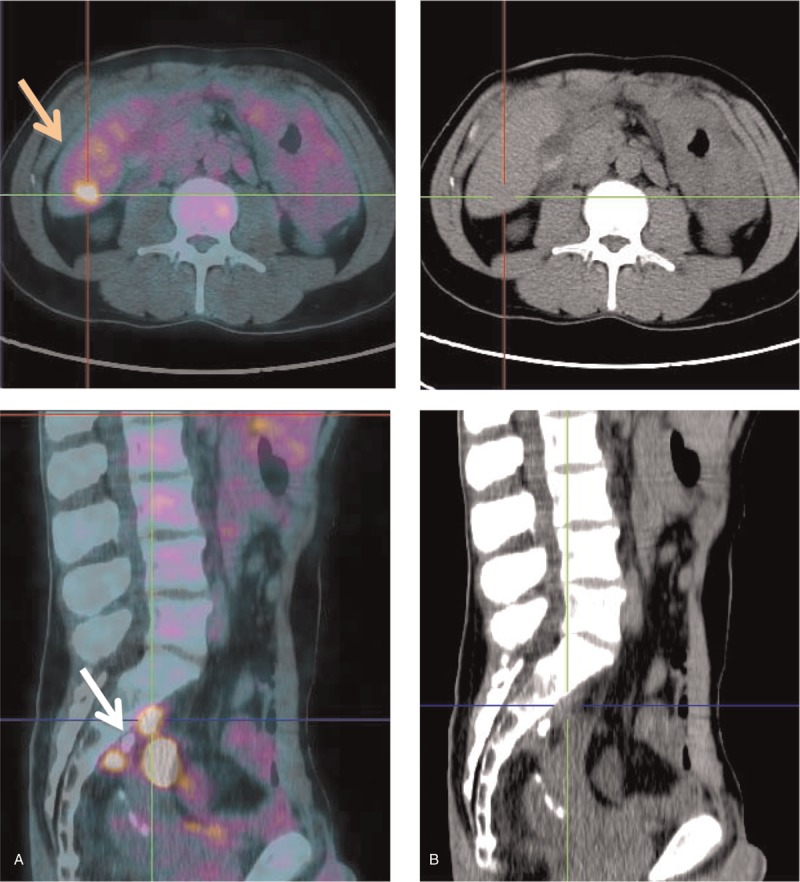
Axial (A) and sagittal (B) images: fused FDG-positron emission tomography/CT (left), CT alone (right). (A) New hepatic metastatic lesions (orange arrow). (B) Tumor implants (white arrow). CT = computed tomography, FDG = fluorodeoxyglucose.
